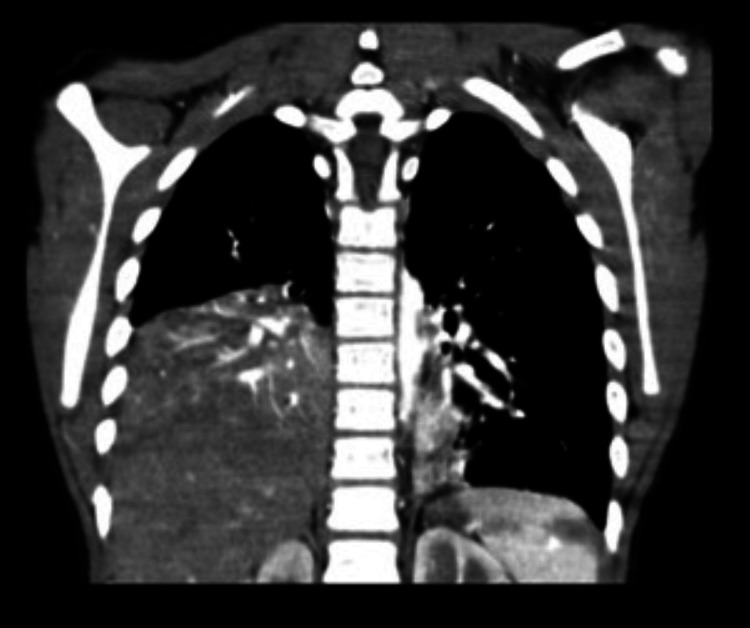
Chest CT showing bilateral posterior-basal consolidative opacities, more on the right side. CT: computed tomography
